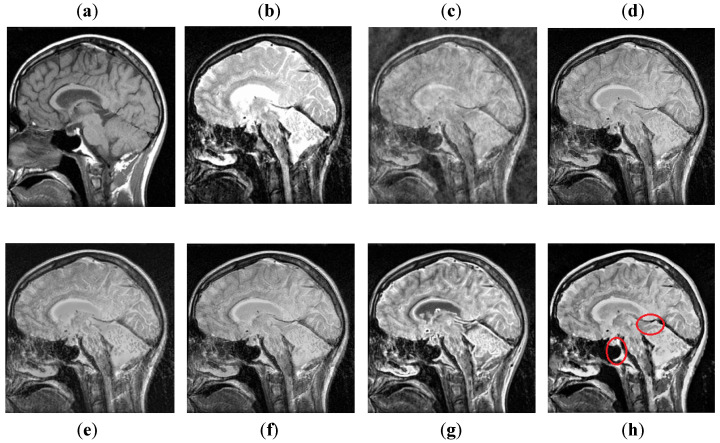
Data-6: (a) CT image, (b) MRI image, (c) DCT, (d) DWT, (e) NSST-SFT-PCNN, (f) CSR, (g) CNN, and (h) Proposed.
